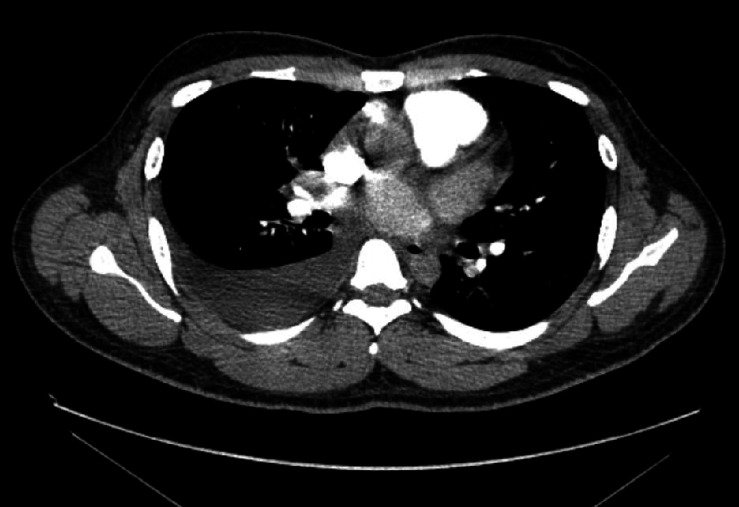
Transverse section of a CT angiogram chest showing a moderate-sized pleural effusion on the right side.
